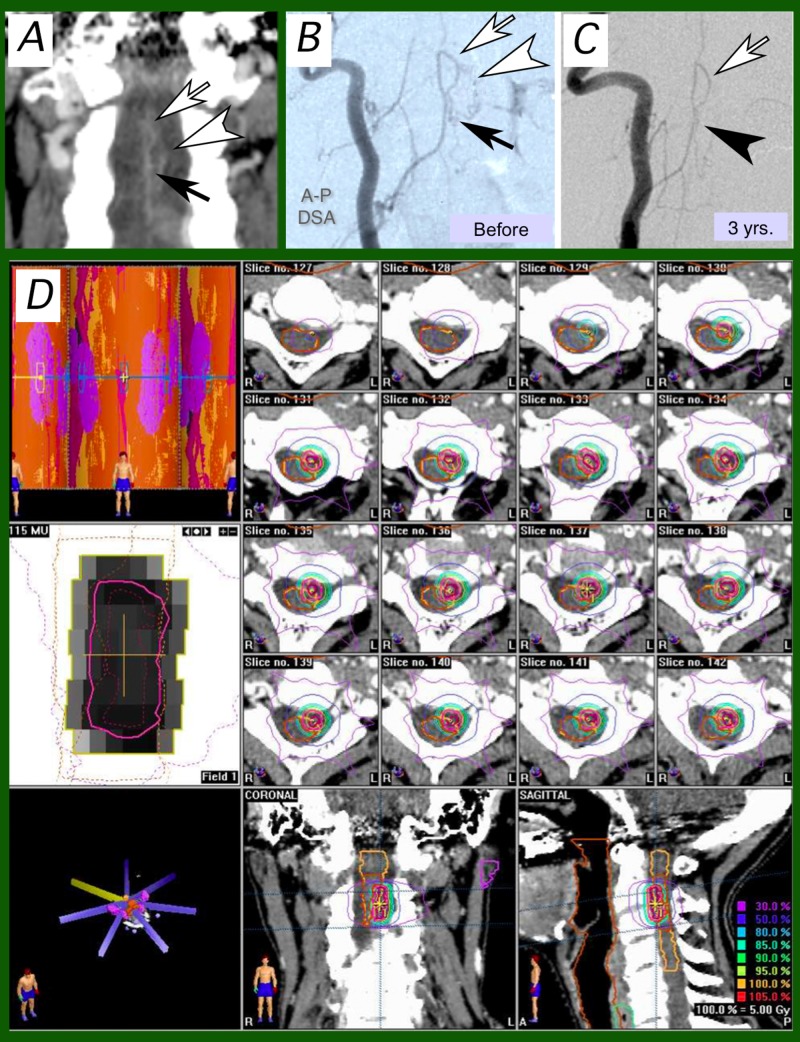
Case 2 2A: Pre-stereotactic radiotherapy (SRT) coronal image of CT and (2B) frontal views of the right vertebral angiography before and (2C) three years after SRT. A little longitudinally long and narrow nidus (arrows, 2A, B) was disclosed and a proximal artery loop (white arrows, 2A-C) and draining veins (white arrowheads, 2A, B) connected to the venous plexus was observed on pre-SRT angiography (2B). 2C: the nidus disappeared, and early venous filling was not observed (arrowhead) three years after SRT; 2D: dose-planning of SRT on BrainSCAN workstation. Seven-beam intensity modulated-SRT plan was employed. CT: computed tomography
